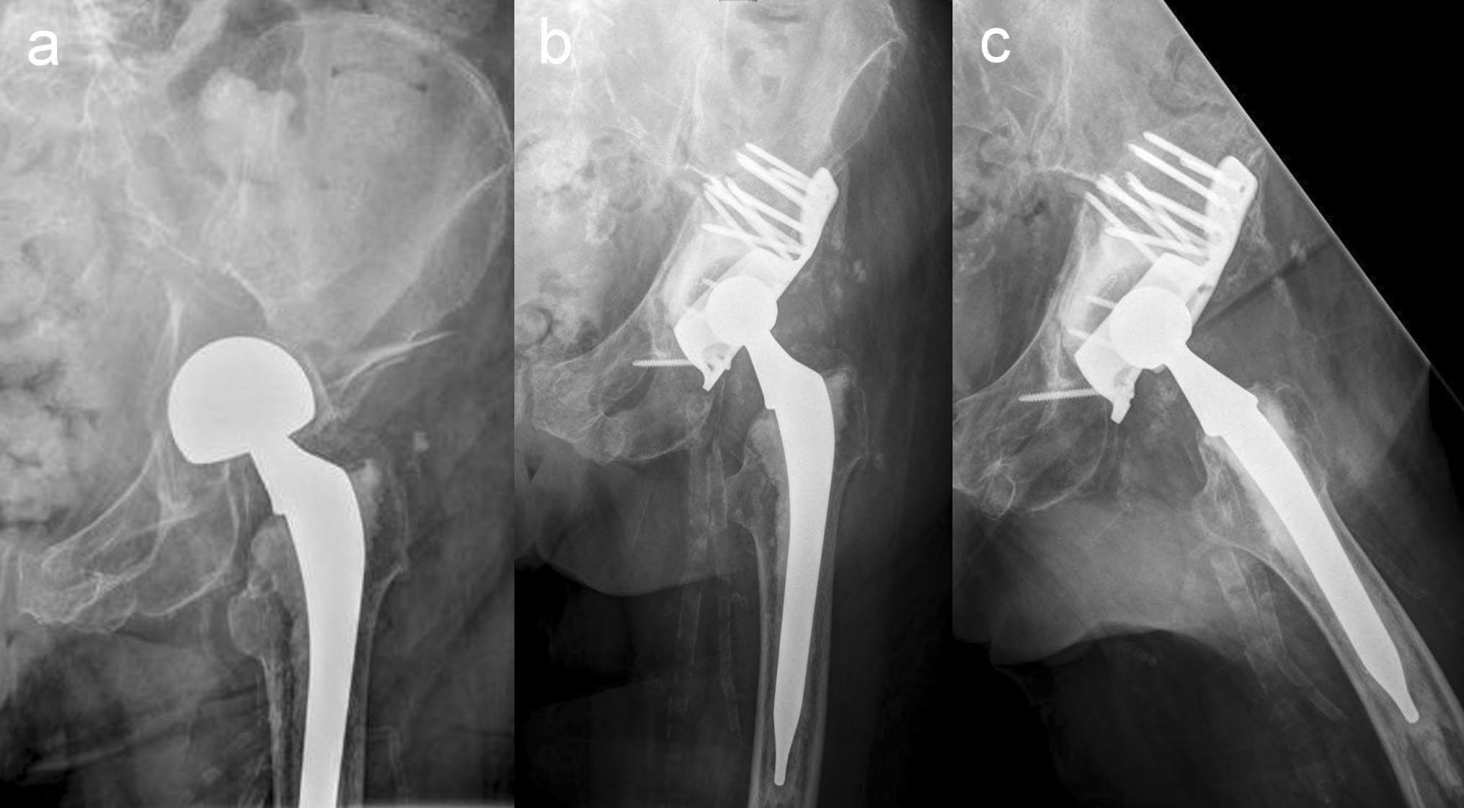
a X-ray of a periprosthetic acetabular fracture. b Postoperative X-ray of the hip joint with implantation of ARRP and THA. c After 6 months fracture healed without bone grafting due to the lack of femoral head
